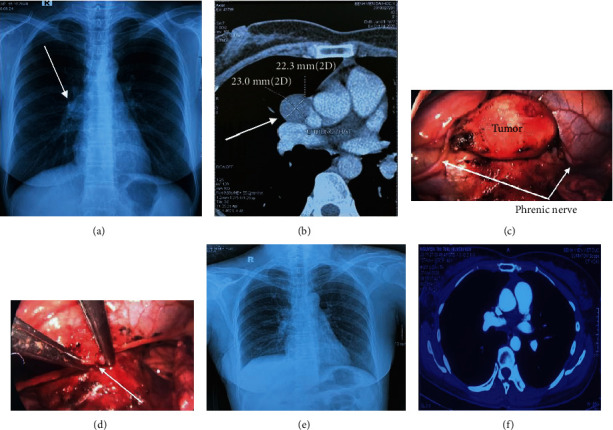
Preoperative, intraoperative, and postoperative images: (a) preoperative chest X-ray (arrow); (b) the middle mediastinal tumor (arrow); (c) intraoperative tumor and the right phrenic nerve (arrow); (d) removed tumor image (arrow); (e) postoperative chest X-ray; (f) the chest CT scanner 1 month after surgery.
